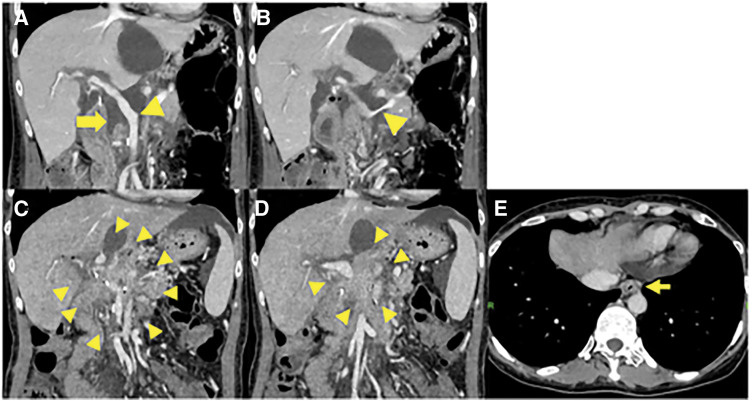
(A, B) Enhanced CT at 1 week after surgery. Enhanced CT showed fluid accumulation around the PV and SV (arrows). There was mild stenosis but no obvious blood flow obstruction in the PV or SV (arrowhead). (C–E) Enhanced CT at 2 months after surgery. (C, D) Inflammatory changes around the PV and obstruction of the SV were observed (arrowheads). (E) No development of esophageal varices (arrow). PV, portal vein; SV, splenic vein
